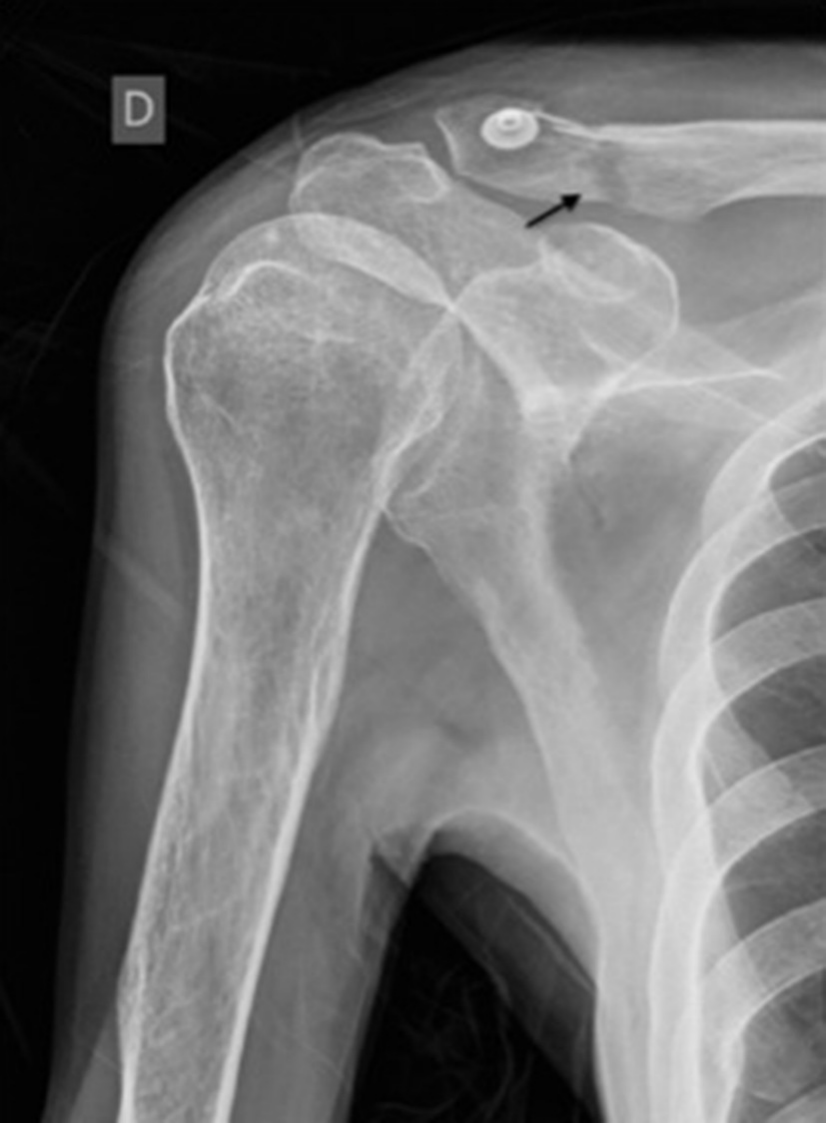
X-ray image of the right shoulder. A wide, lucent fracture line without dislocation between the fragments is seen at the distal third of the right clavicle (black arrow). The X-ray was taken during the patient’s first visit to the emergency department and was misinterpreted as normal.
